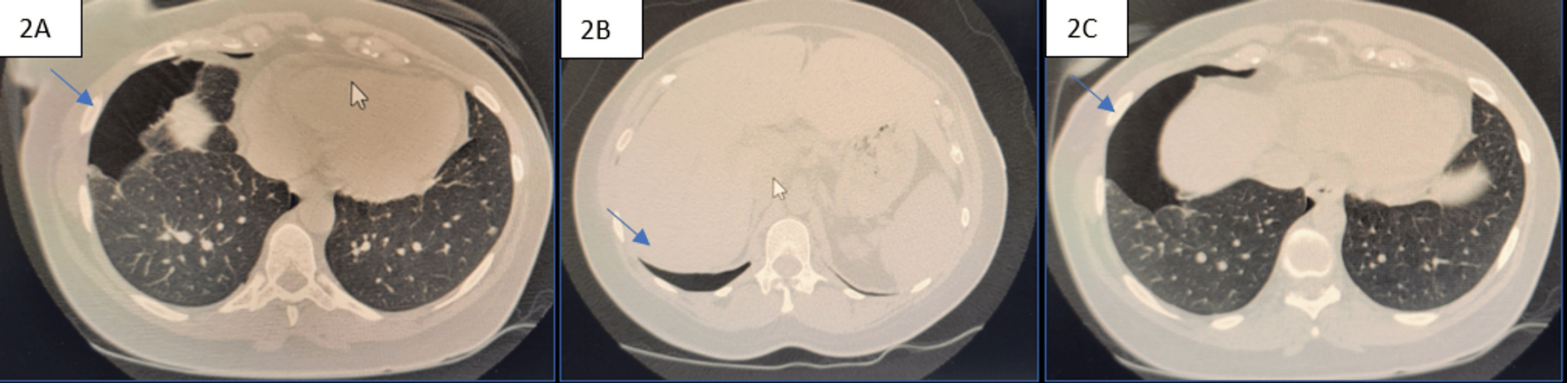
Non-contrast chest CT. 6/19/24 at 3:34 am: loculated right pneumothorax in the mid-lower lobe regions with a maximum thickness of 4 cm, associated with adjacent parenchymal collapse. A small loculated pocket of pneumothorax in the right apical region with underlying pneumo-atelectasis. Tiny subpleural parenchymal nodule in the left lower lobe.
